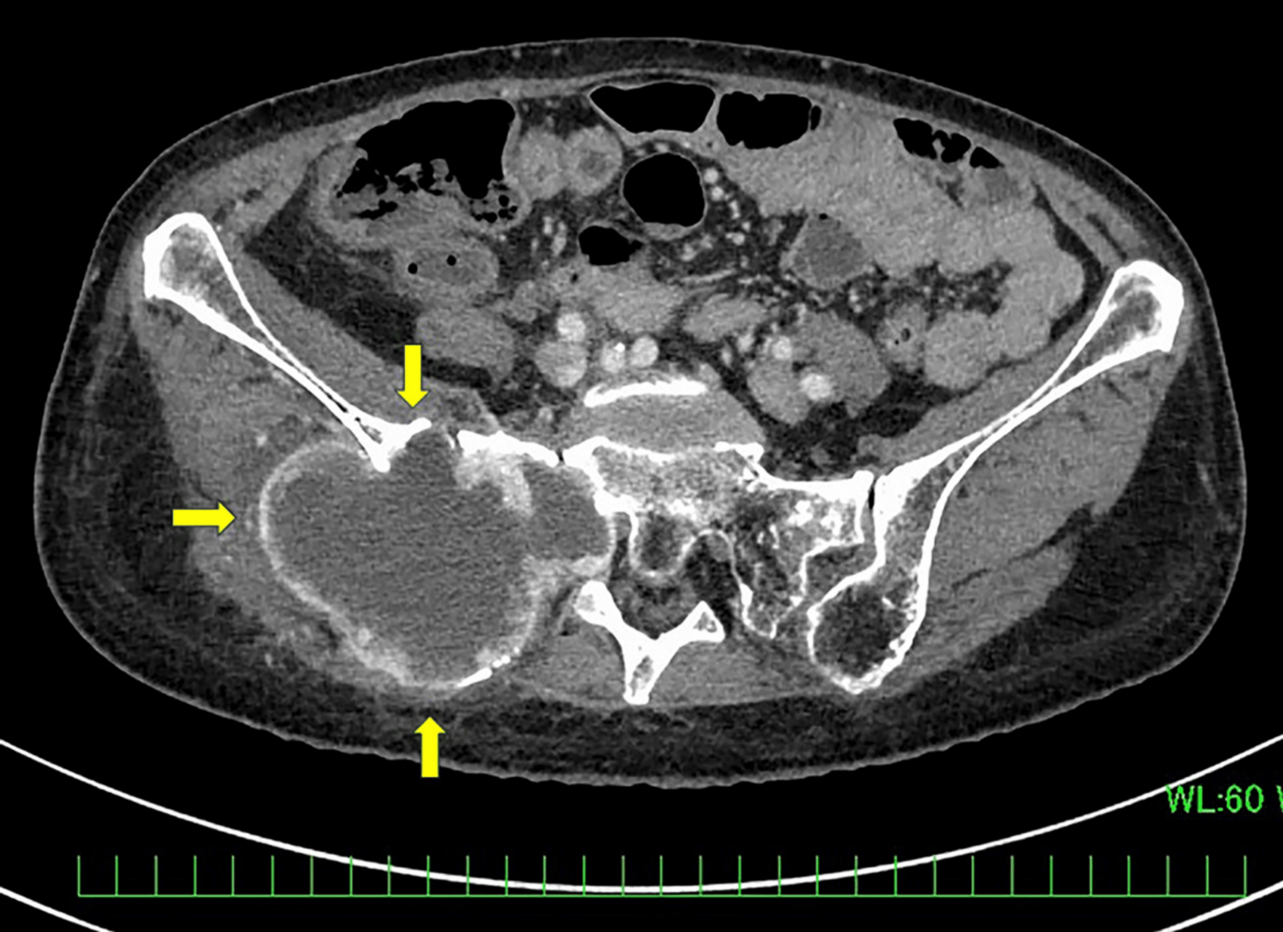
Follow-up imaging of Case 1 after selpercatinib therapy. Contrast-enhanced CT showing a decrease in the size of the right pelvic bone metastasis compared with baseline imaging (arrows).
